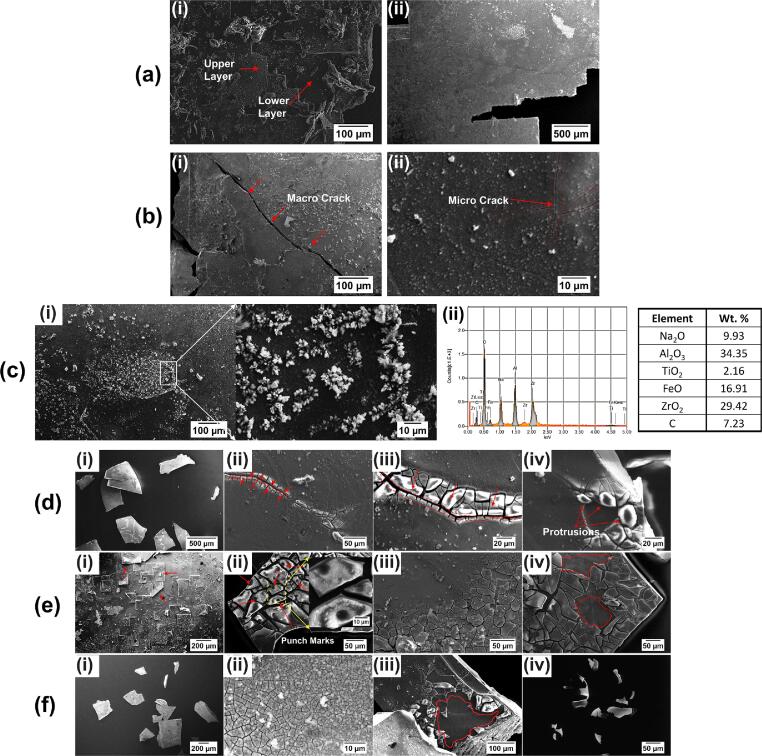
(a) SEM images of an extracted Al_3_Zr crystal showing layered and faceted morphology, (b) presence of pre-existing micro-cracks, and (c) oxides and the EDS spectrum with elemental composition. (d) SEM morphological images of cavitation damage induced after 3 sec, (e) 6 sec, and (f) 9 sec sonication, obtained at ultrasonic amplitude of 210 μm peak-to-peak.
